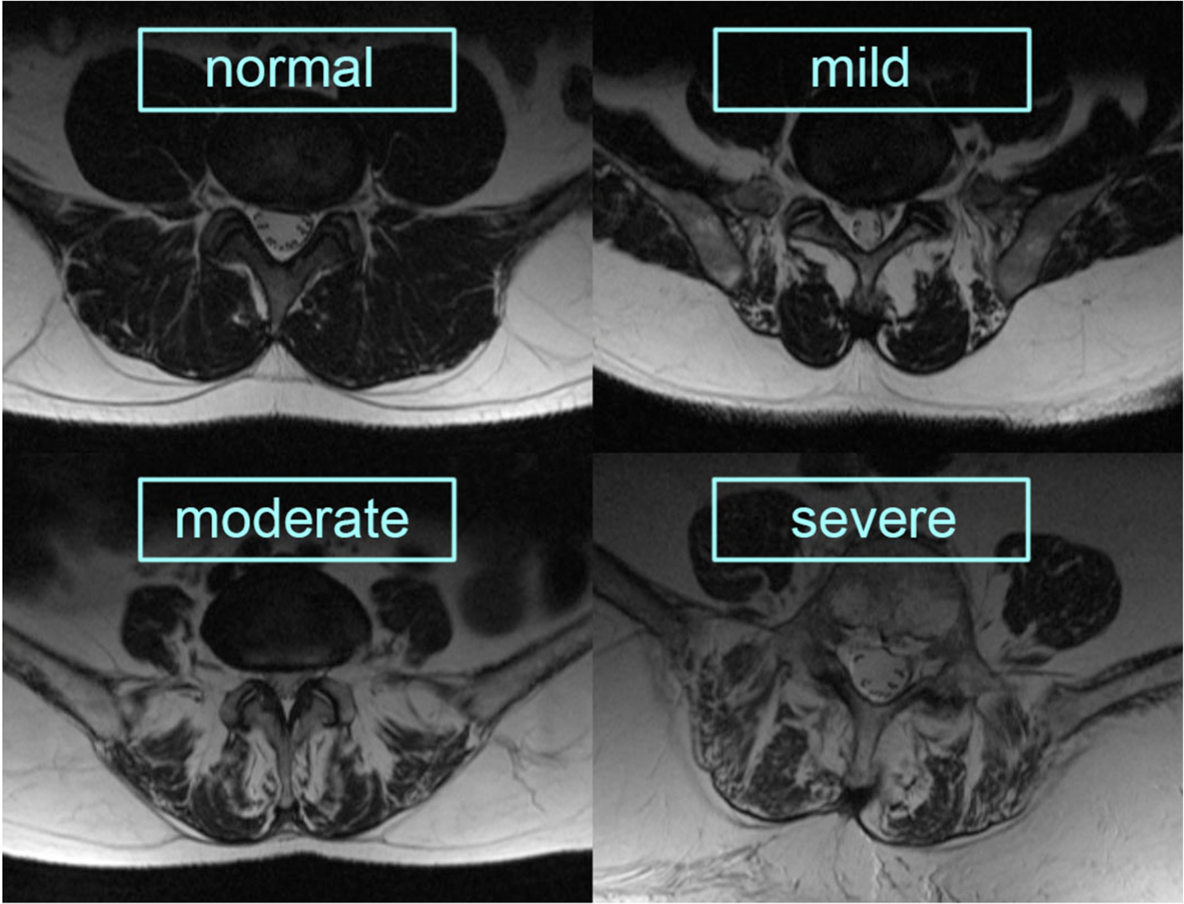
Stages of fatty infiltration of the LMM on T2-weighted axial MRI scans
